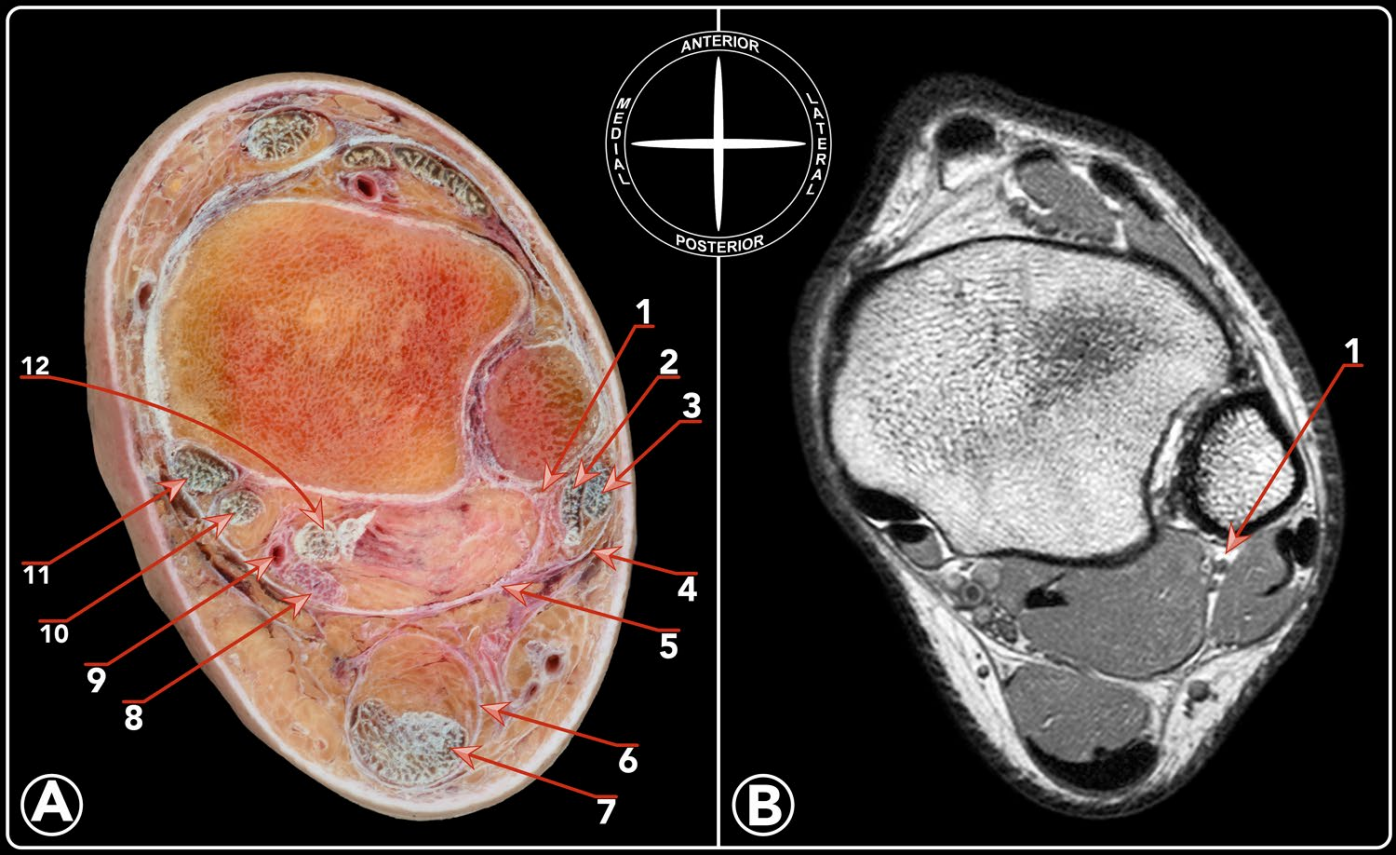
a Cross section at the level of the tibial pilon. b Comparative MRI to highlight the location of the PFTCLC. 1. PFTCLC. 2. Peroneus brevis tendon. 3. Peroneus longus tendon. 4. Superior peroneal retinaculum. 5. Superior lamina of the PFTCLC. 6. Fascia of the superficial posterior compartment. 7. Achilles tendon. 8. Tibial nerve. 9. Posterior tibial artery. 10. Flexor digitorum longus tendon. 11. Tibialis posterior tendon. 12. Flexor hallucis longus tendon
